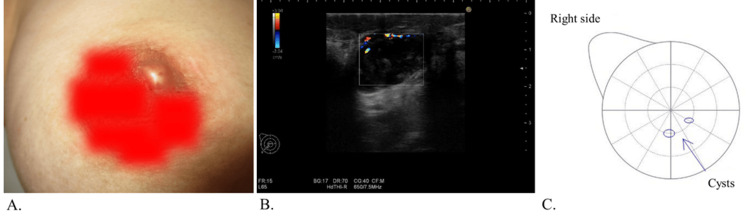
(A) Abscess formation around the right nipple. (B) Ultrasonography of the right nipple revealed abscess formation with a blood flow signal. (C) Ultrasonography imaging around the right nipple after drainage and antibiotic administration showed no abscess formation, but several existing cysts.
